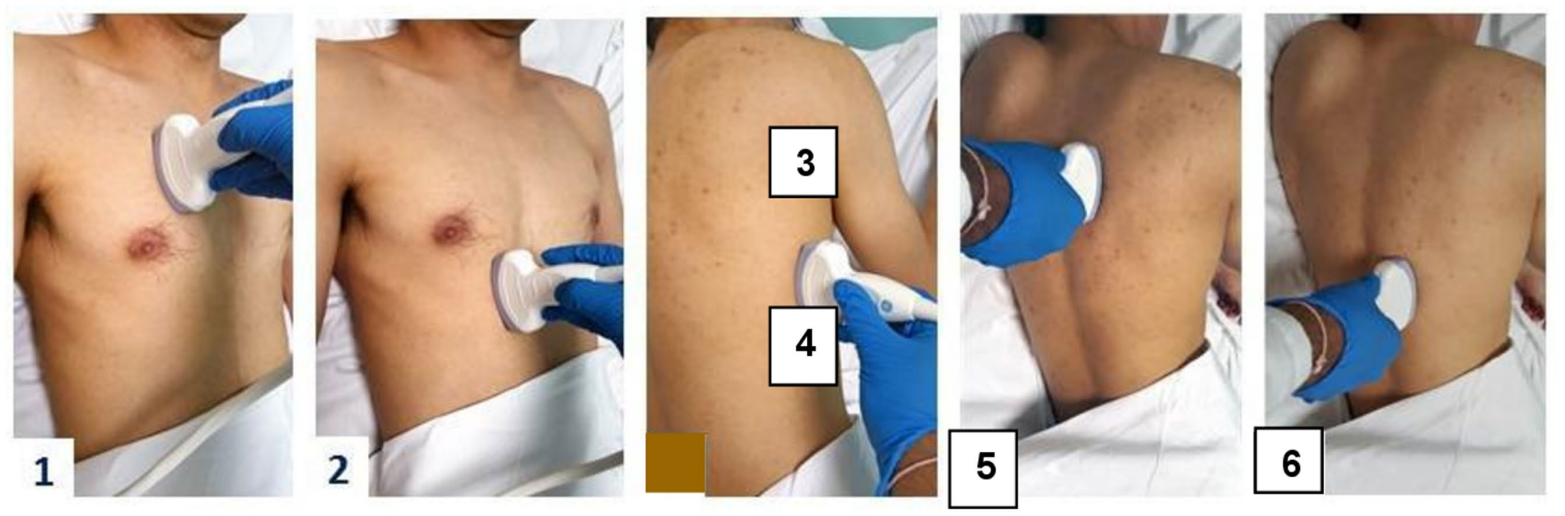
Six-zone/hemithorax approach. For lung ultrasound examination, we chose the approach of six zones per hemithorax. Each hemithorax was divided into three regions: anterior, lateral, and posterior. Each region has an upper and lower quadrant, i.e., six quadrants per hemithorax and twelve quadrants in total.
